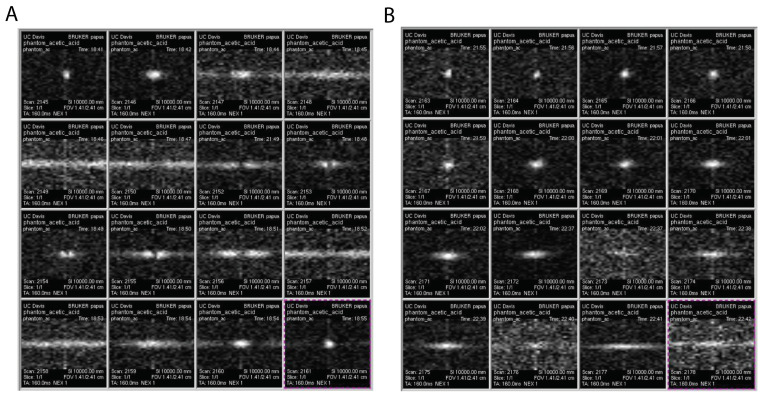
Diamond MRI with different flip angles. (A) The pulse durations are 5, 10, 15, ..., 80 μs respectively for each image. We can determine that optimal pulse duration should be within 20 μs. (B) The pulse durations are 4, 5, 6, ..., 19 μs respectively for each image. The text on each image is the frame number, time when the images are taken, and FOV (1.41 × 2.41 cm).
